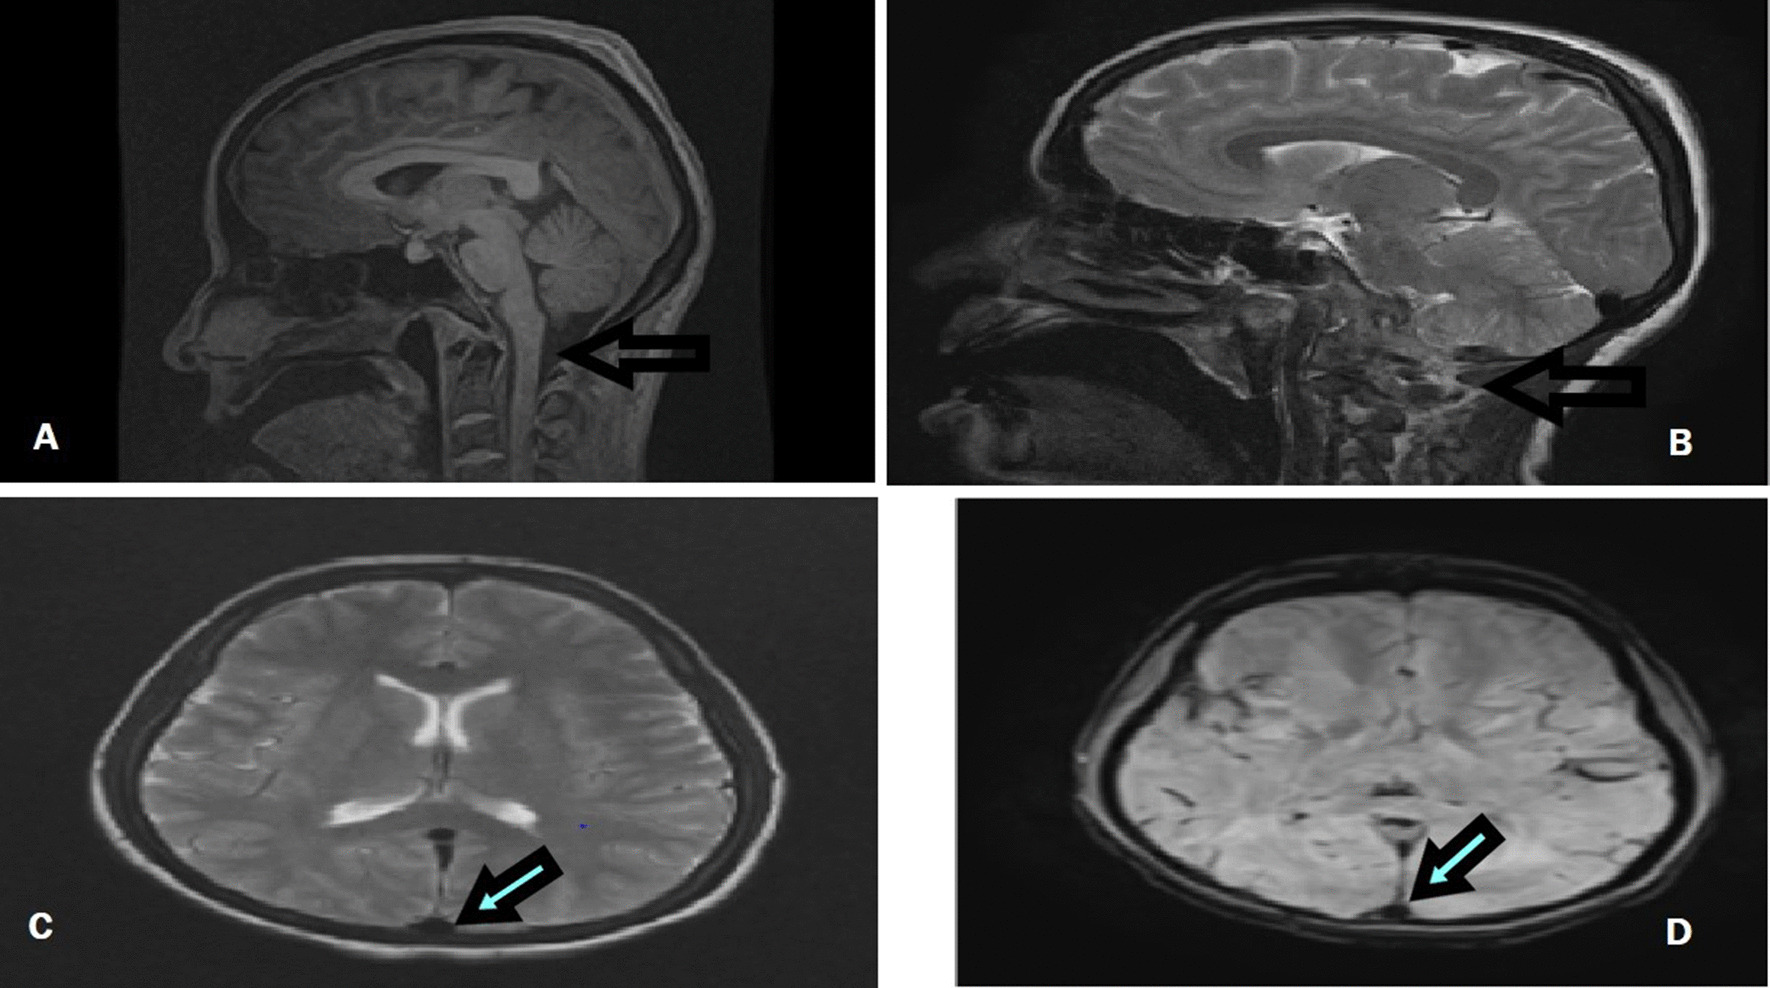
MRI brain showing tonsillar herniation via black arrows on sagital plane (A, B) and compression of ventricle with slight deviation of cereberum to left is shown via arrows on axial plane (C, D)
